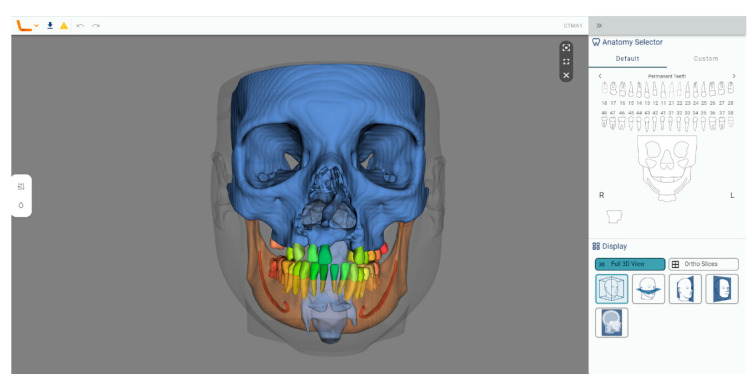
Relu’s user interface (CT w/A 1 displayed).
